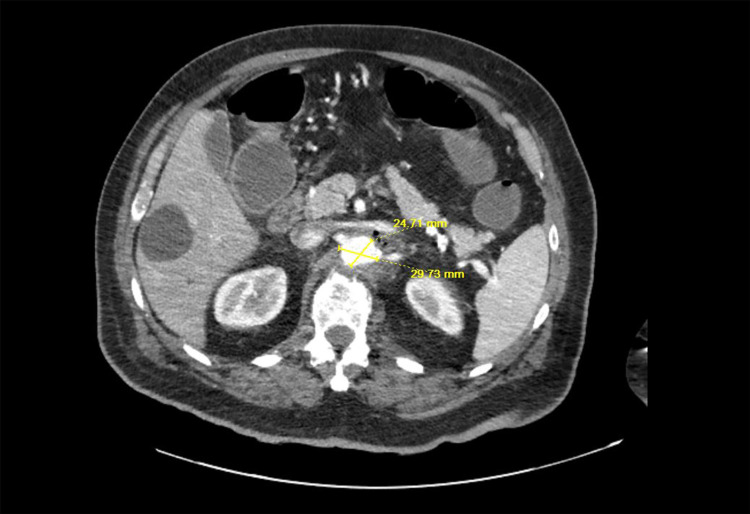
Computed tomography angiography of the abdomen showed mid abdominal aortitis
